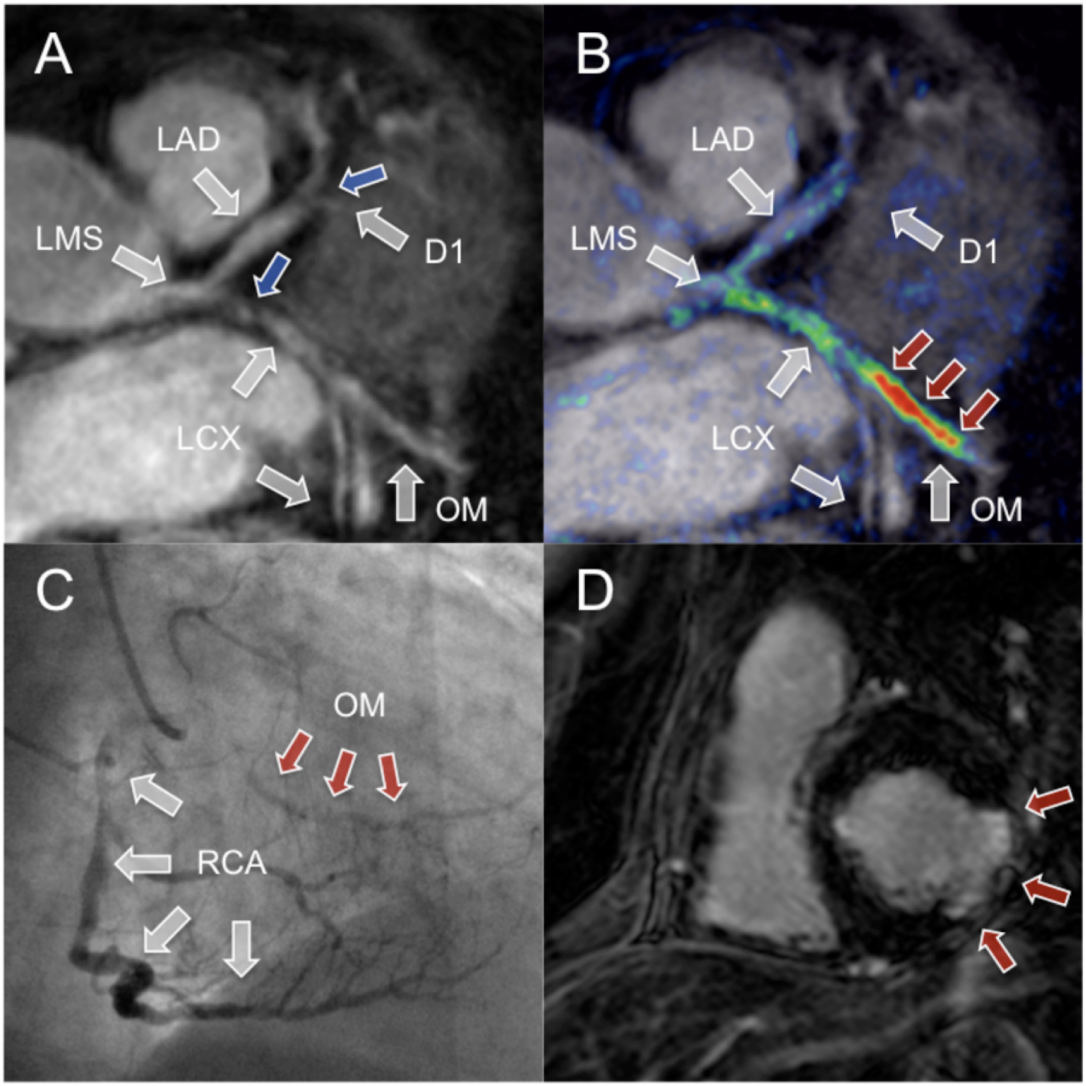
CE-MRCVI findings with troponin positive ACS in the LCX. Coronary MRA (A) with troponin positive ACS showed decreased vessel lumen size of the proximal LCX and first marginal branch (grey arrows). To highlight the relationship between and morphology (A) and CE-MRCVI, images were fused in a way similar to PET/CT (B). CE-MRCVI displays high signal intensity (red arrows) within the first marginal branch. Corresponding XCA (C) confirmed CE-MRCVI findings with mid LCX lesion. Short axis delayed enhancement scan shows predominantly transmural myocardial infarction of the mid-ventricular infero-lateral segment. MRA: magnetic resonance angiography, CE-MRCVI: contrast enhanced magnetic resonance coronary vessel wall imaging, PET/CT: positron emission tomography/computed tomography.
